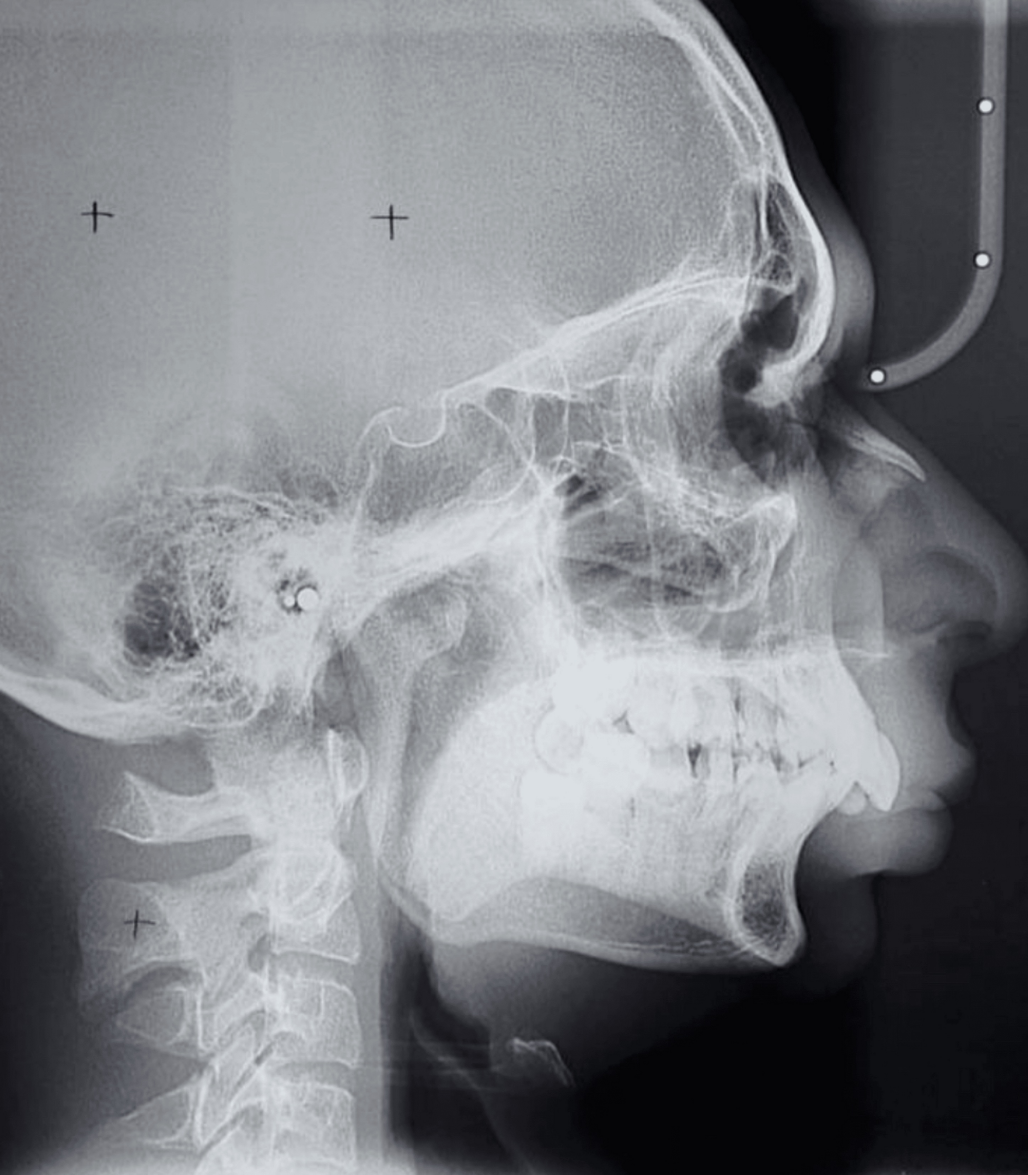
Preoperative lateral cephalometric radiograph of the patient
